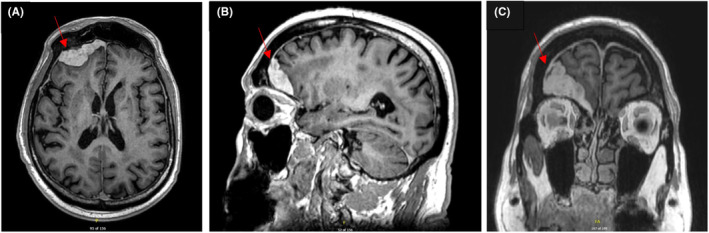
Oct 17, 2016 (Pre–op). MRI head, post gadolinium 3‐D T1 axial and sagittal of primary dural Hodgkin lymphoma. Right frontal dural mass visualized with vasogenic edema in the right frontal white matter.
